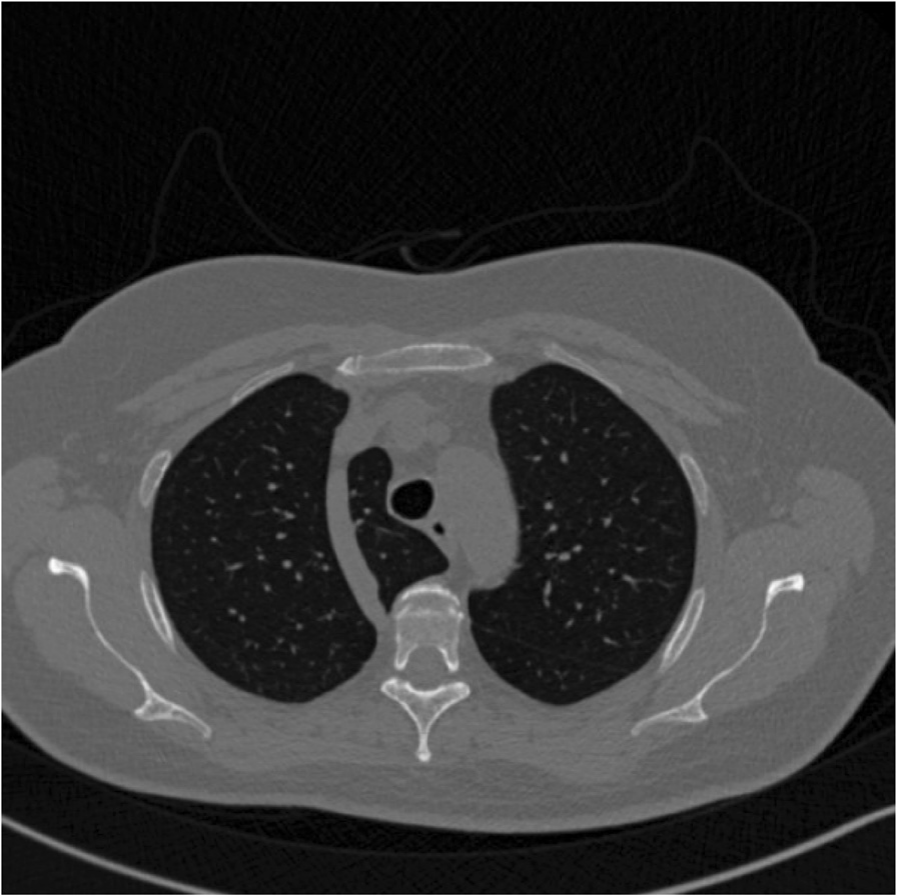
Postoperative chest CT scan. A chest CT scan showing diffuse, small, thin-walled cystic lesions in the parenchyma.
